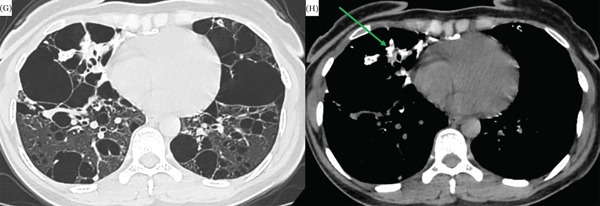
Cystic amyloidosis. (G) Axial chest CT (lung window) showing multiple thin‐walled cysts causing extensive parenchymal destruction. (H) Mediastinal window demonstrating a calcified nodule adjacent to the cysts (green arrow), representing a mixed radiological pattern.
